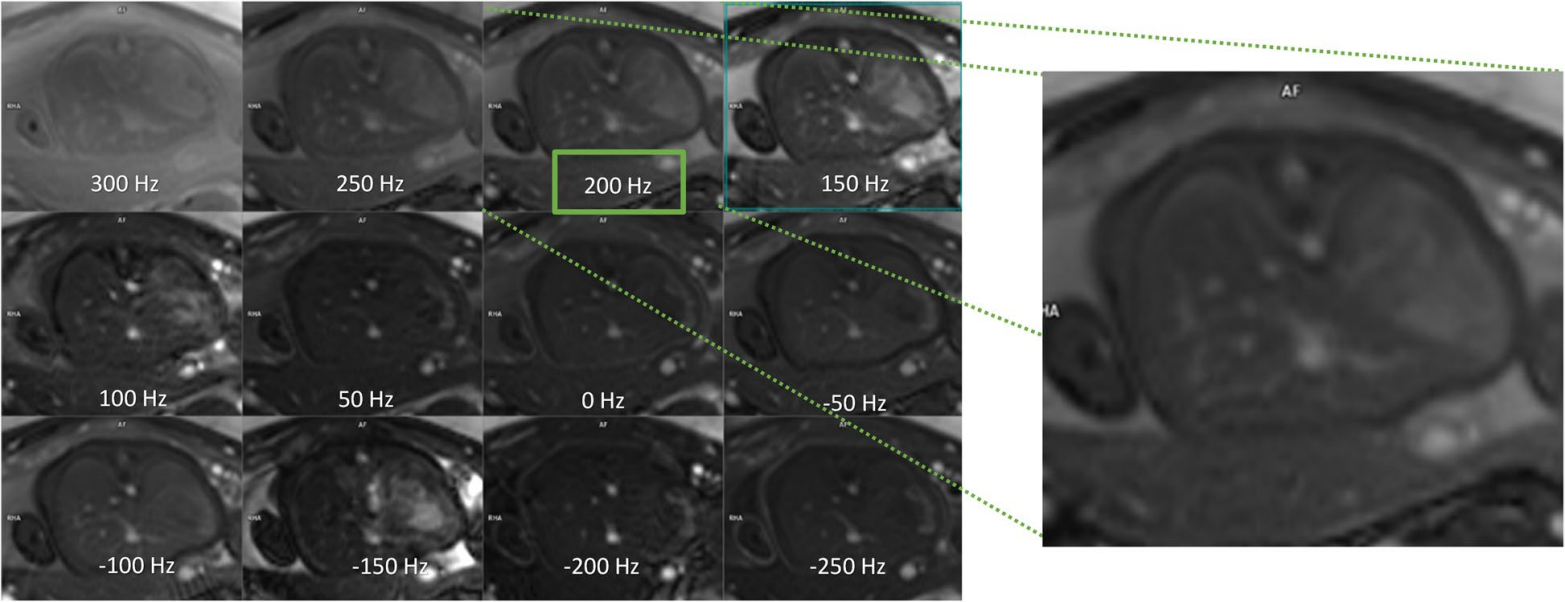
Single-slice transversal views of the “frequency scout” with twelve different offset frequencies. The frequency scout is planned in one or a few slices around the organ of interest. The “best” frequency is chosen by the operator and/or radiologist depending on the preferred contrast and/or the absence of the banding artifact around the point of interest, and the actual T2-weighted balanced steady-state-free precession is acquired
